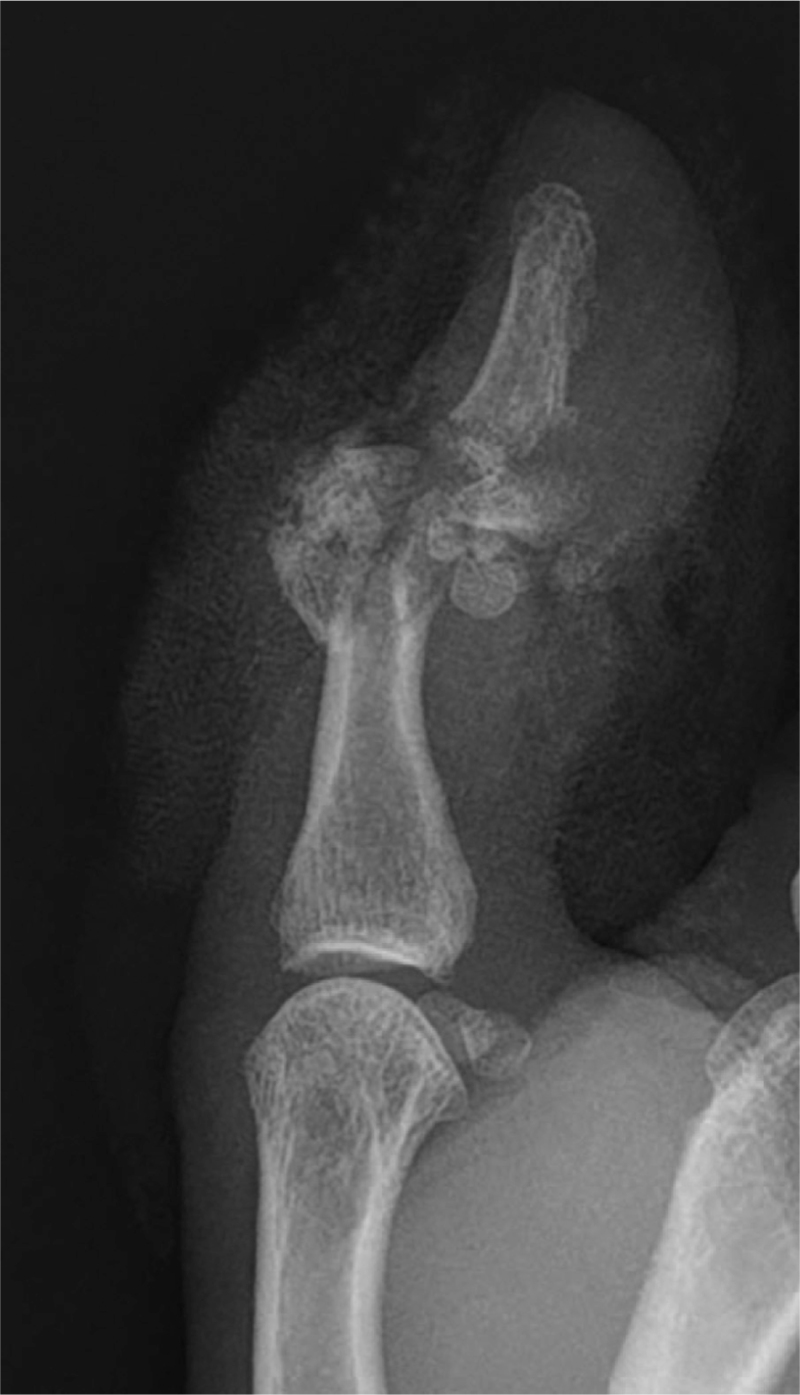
Preoperative x-ray of the affected finger.
